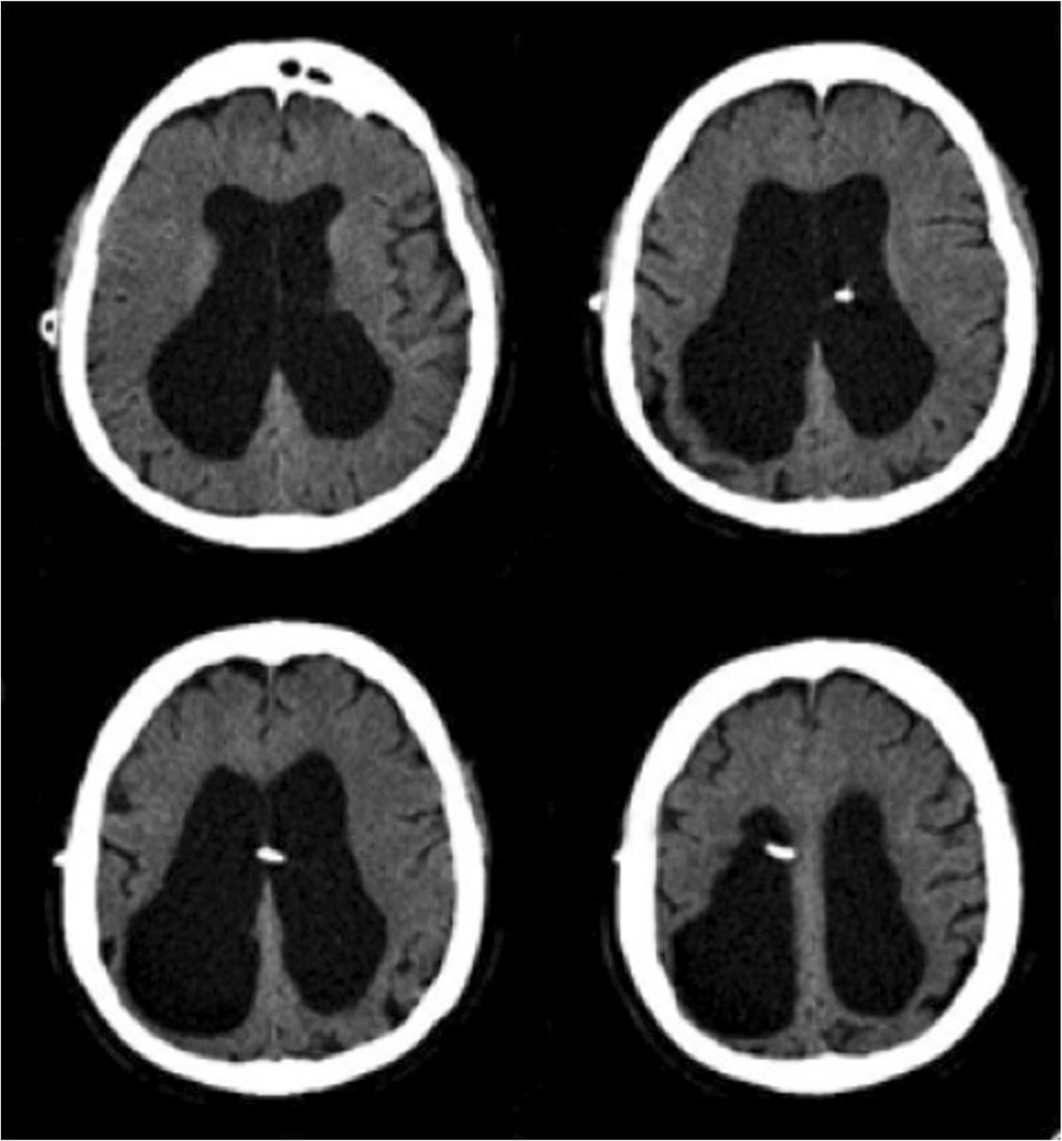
Computed tomography scan one year after subdural hematoma. The computed tomography scan performed one year after subdural hematoma shows resolution of the subdural hematoma and dilated ventricles.
